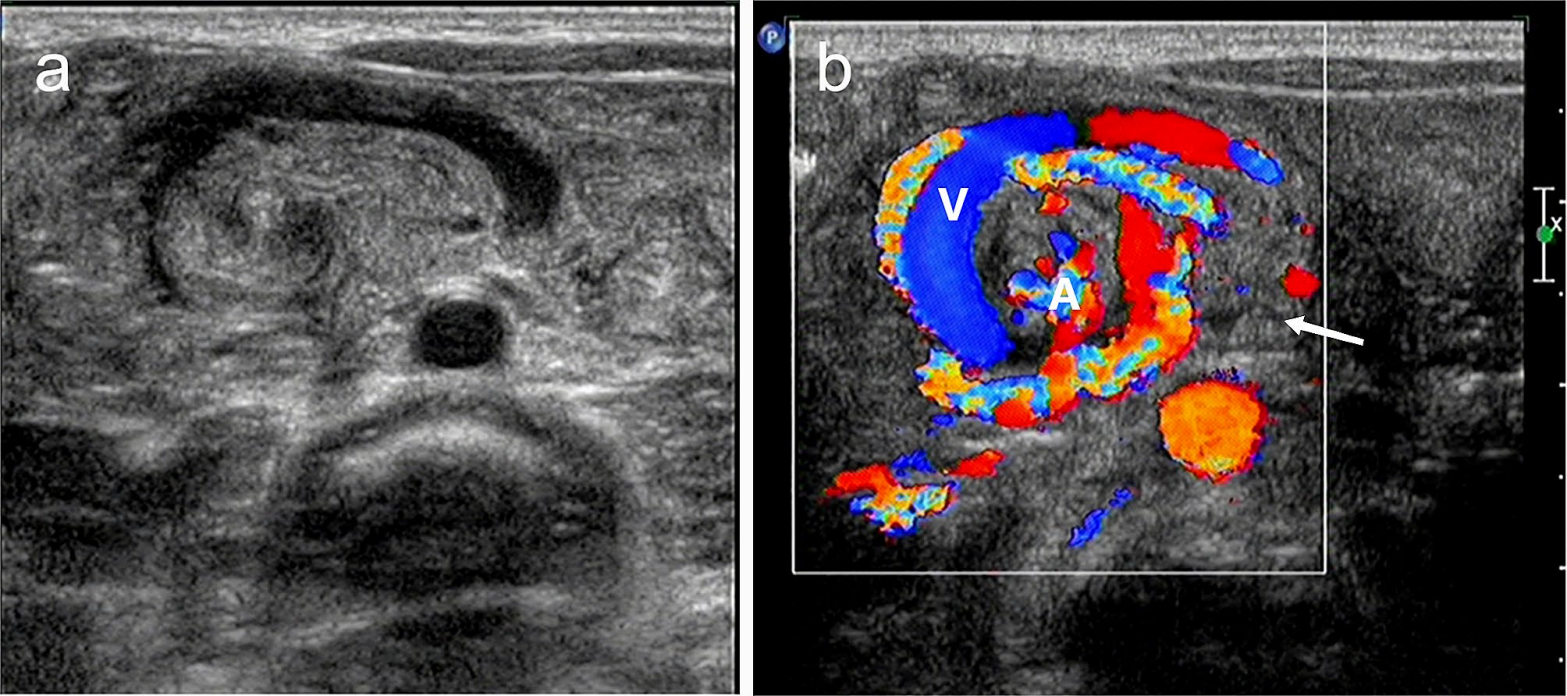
Representative imaging scans of a 2-year-old male patient presenting vomiting and abdominal pain. A: Congenital malrotation of the intestine with midgut volvulus was observed. The cross-sectional color doppler ultrasound image visualizing a clockwise twisting of the superior mesenteric vein (SMV) around the superior mesenteric artery (SMA) at the center of the intestinal twist, and a target ring of an isoechoic mass (white arrow). B: CDFI showed a swirling sign centered on the SMA with red and blue blood flow signals. Congenital malrotation of the intestine with midgut volvulus was postoperative confirmed. The greater omentum and the distal mesentery were tightly adherent to form a bundle of intraperitoneal hernia.
